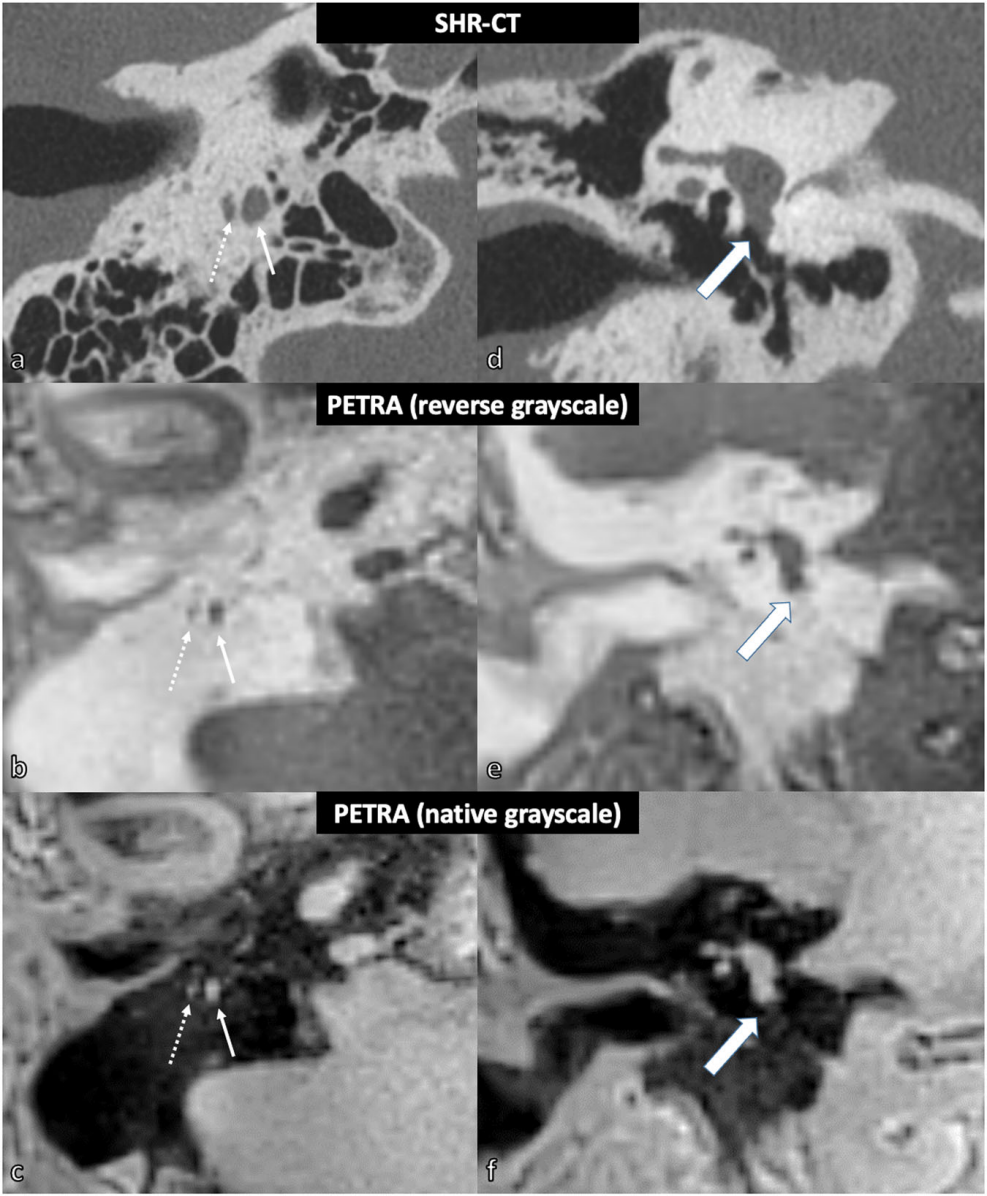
Right mastoid portion of the facial nerve (MFN), bony segment of the chorda tympani nerve (CTN), and round window niche with SHR-CT and PETRA MRI sequences (example in living patient). The mastoid portion of the facial nerve (MFN: solid white arrow) and the origin of the bony segment of the chorda tympani nerve (CTN: dotted white arrow) are clearly visible in SHR-CT (a) and PETRA MRI sequence with inverted grayscale (b) and native grayscale (c). The round window niche (large white arrow) is clearly visible in SHR-CT (d) but remains indistinguishable in the inverted (e) and native (f) grayscale PETRA MRI sequence. MRI, Magnetic resonance imaging; SHR-CT, Super-high-resolution computed tomography
